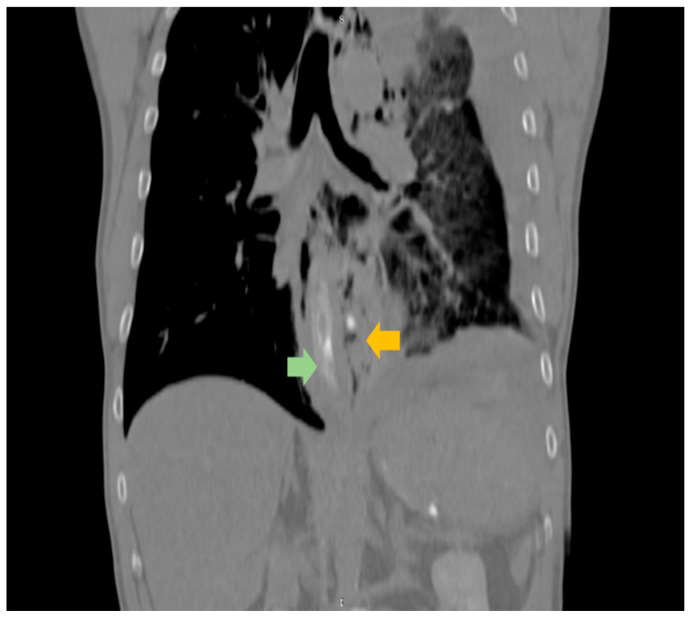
Longitudinal CT sections obtained after oral contrast administration demonstrated extraluminal leakage of the contrast material into the mediastinum at the distal esophagus level. The distal esophageal segment appeared thickened and edematous (green arrow), and the site of perforation was visualized as the point of contrast escape from the esophageal lumen (orange arrow).
